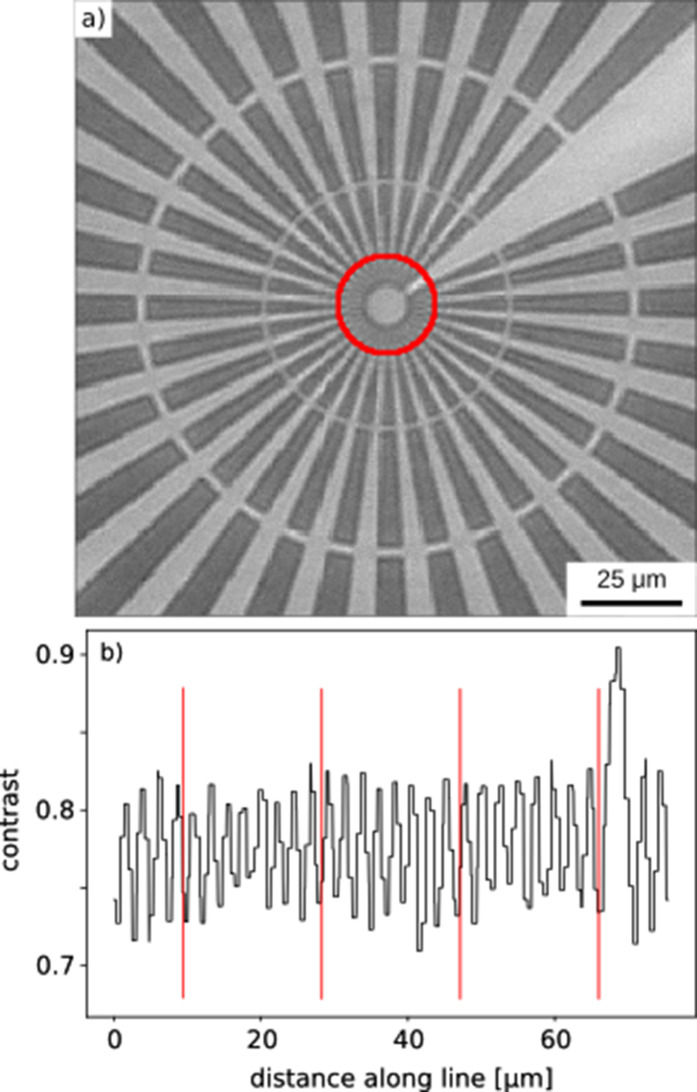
Features of 1.2 µm size can be resolved with the imaging camera setup. (*a*) High-resolution radiographic projection of a Siemens star (collected with monochromatic beam at 15 keV); the red circle highlights a radius of 12 µm around the Siemens star centre for pixel intensity measurement. (*b*) Pixel intensity along the red circle, with ∼8% difference between high- and low-contrast regions.
